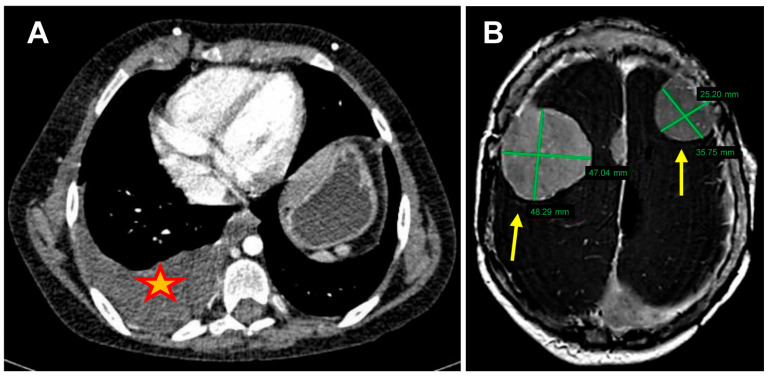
(A) Computed Tomography scan of abdomen (transverse plane), showing pleural thickening and effusion (yellow star); (B) brain MRI (T1 post-contrast, axial), showing rapidly increasing bilateral recurrent meningiomas (yellow arrows).
